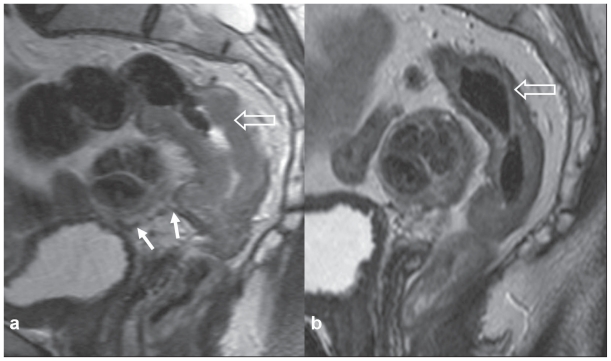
Patient 2: Sagittal T2 weighted fast spin echo (TR3900 TE 120) MRI scans of a) pre-chemoradiotherapy, showing a large rectal tumour (open arrow) arising entirely above the peritoneal reflection (arrowed) into the sigmoid colon, almost to the level of S1/2, and b) considerable tumour regression and downsizing 4 weeks post-chemoradiotherapy, with extensive low signal intensity indicating fibrosis (open arrow), consistent with a radiological complete response.
